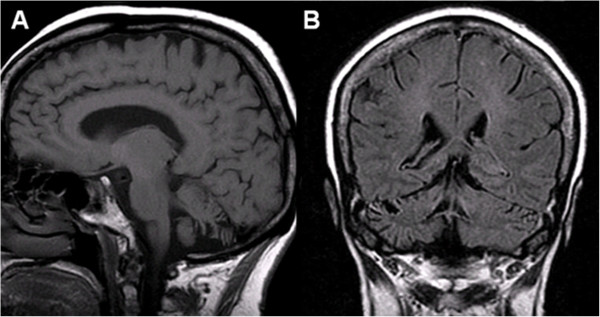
Magnetic resonance imaging of the brain, patient 1. Sagittal (A, T1 weighted) and coronal (B, FLAIR T2 weighted) MRI showing cortical atrophy in the cerebellar hemispheres and the inferior vermis with enlargement of the cerebellar sulci, the fourth ventricle and the inferior cerebellar cistern, but no atrophy of the cerebral cortex, midbrain, or pons.
